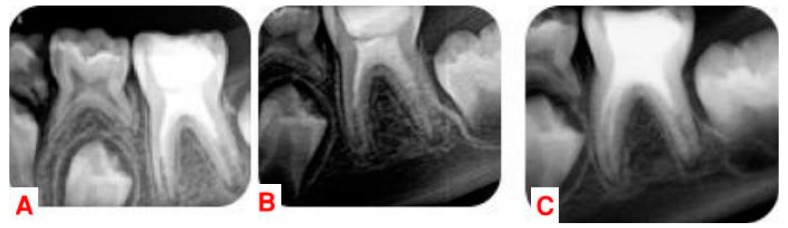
(A) Recall radiograph at three months. (B) Recall radiograph at nine months. (C) Recall radiograph at twelve months.
